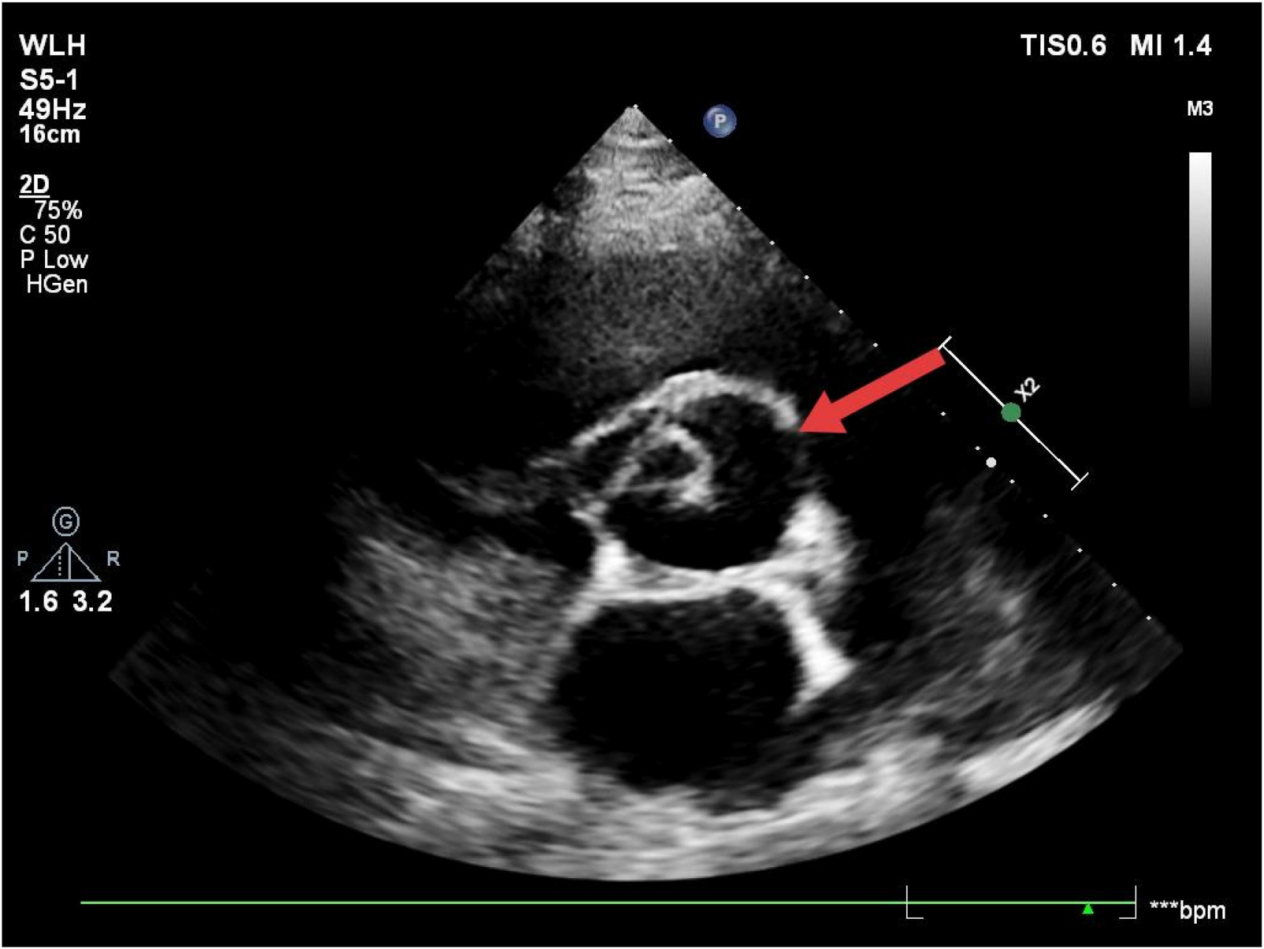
Transthoracic echocardiography (short-axis view of the great arteries). The arrow indicates the aortic valve, which appears to be a unicuspid valve.
